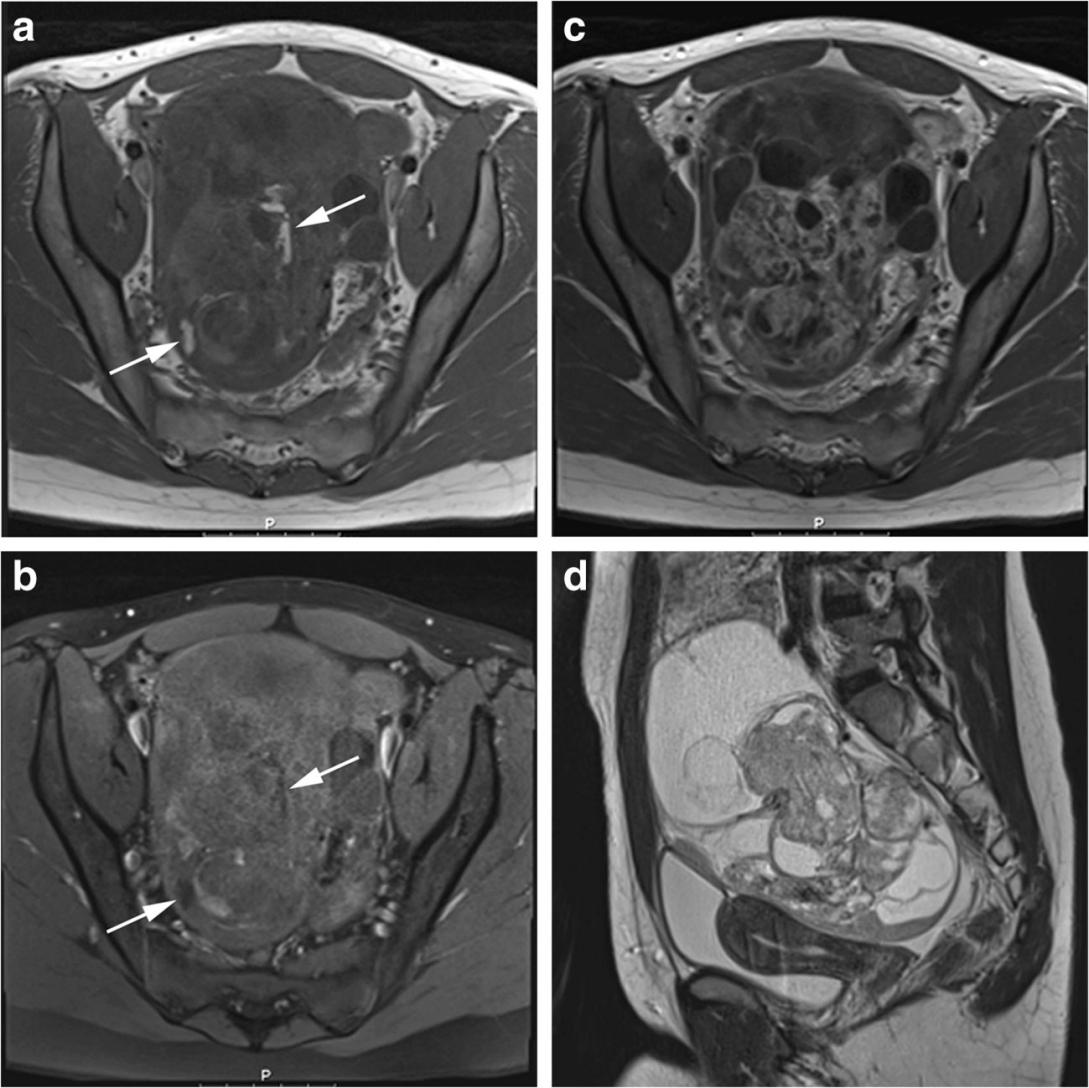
An example of immature teratoma grade 1 of the right ovary in a 15-year-old girl, treated as a benign tumour with local resection and follow-up. Axial T1-weighted before and after administration of gadolinium contrast (a, c), axial T1-weighted with fat-suppression (b) and sagittal T2-weighted turbo spin echo (d) show a cystic-solid mass with fatty components (arrows). Intralesional fat is diagnostic for a teratoma. The relative large amount of enhancing parts increases the risk of immature components
